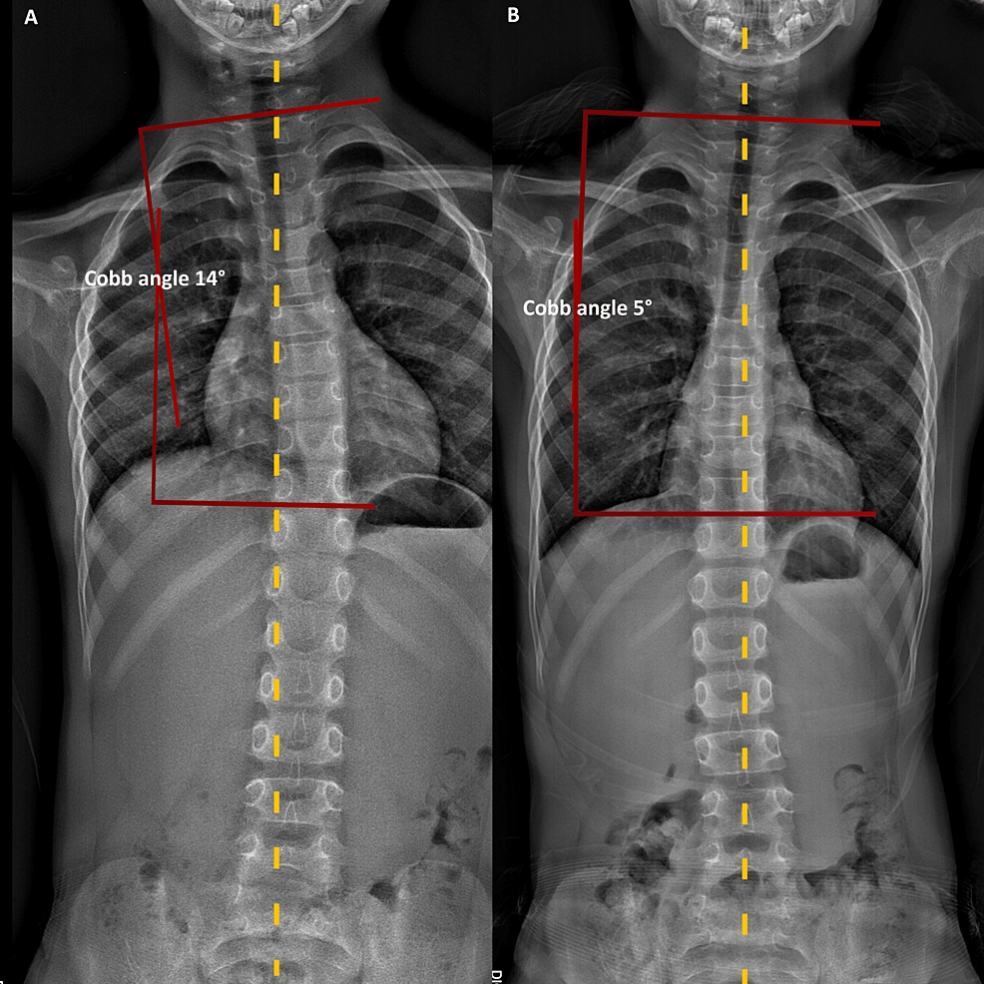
EOS® full-spine radiography in frontal view. (A) Shoulder asymmetry and thoracic scoliosis were present before chiropractic intervention. The central sacral vertical line (yellow line) signifies the global axis. (B) At the nine-month reassessment, the patient showed notable improvements in postural balance. The radiographic parameter showed improvement of Cobb angle measurement from 14° (to the left) to 5° (to the right), and assessment of the central sacral vertical line (yellow line) revealed a considerable improvement in the coronal balance of spinal scoliosis.
